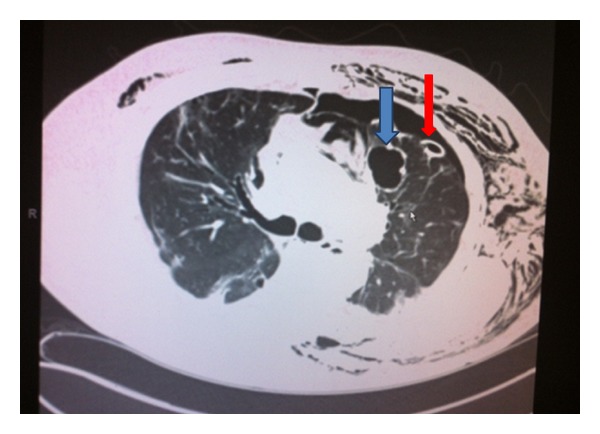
Chest CT with contrast after one month of axitinib that showed left pneumothorax, cutaneous emphysema, thoracic tube (red arrow), and necrotic RCC metastasis in the left lung (blue arrow).
